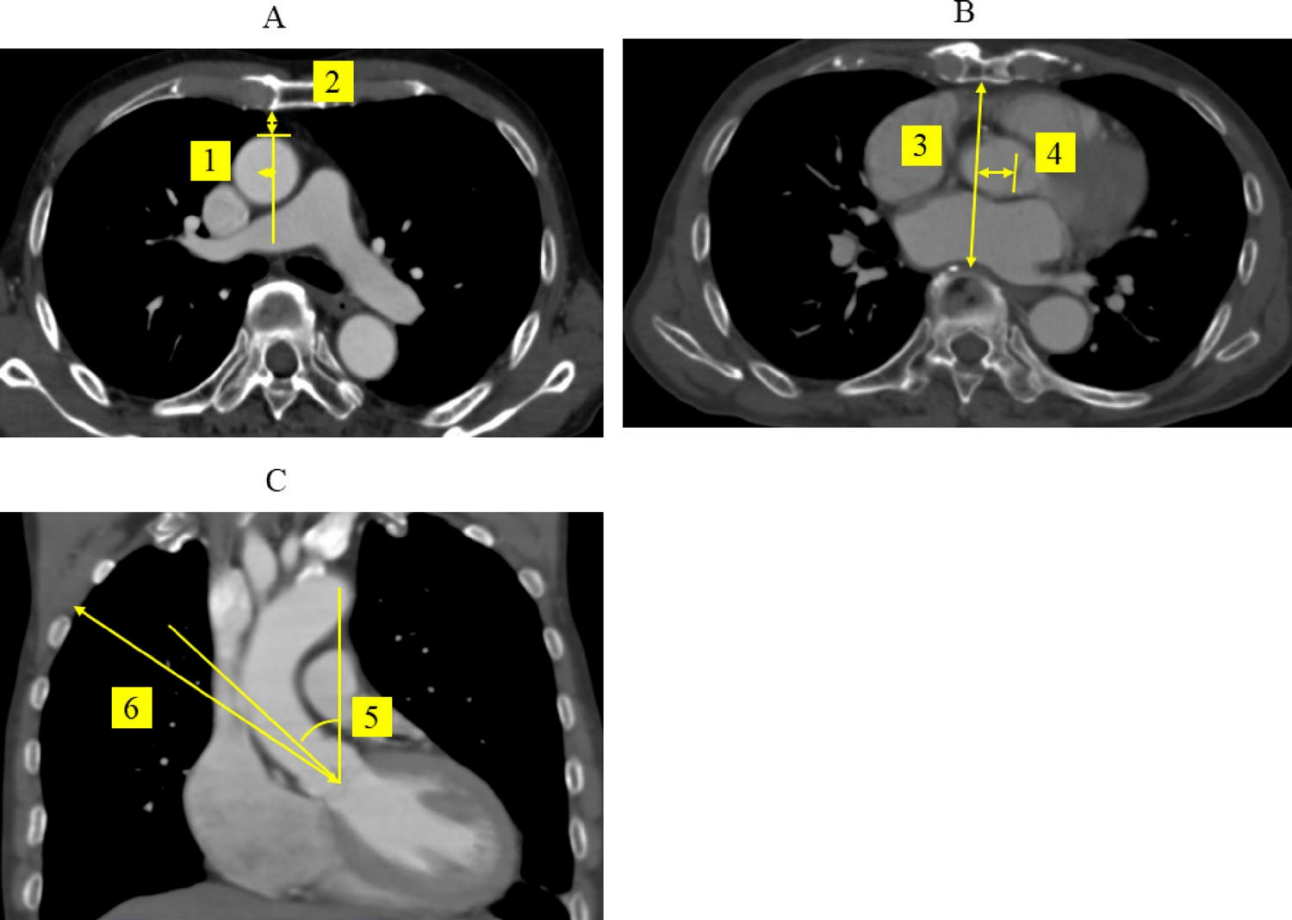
Preoperative CT: (1) laterality of aorta (dextroposition was measured as the distance of the aortic center lateral to the right sterna edge at the same level of the pulmonary trunk on axial image), (2) aorta depth (the distance from the posterior sternal edge to the anterior aortic edge at the level of the pulmonary trunk on axial image), (3) anteroposterior (AP) thoracic diameter (distance from the sternum to the vertebra at the level of the AV at the same level of AV on axial image), (4) AV deviation (distance from the median line to the AV at the same level of AV on axial image), (5) aortic angle (the angle between the ascending aorta and the vertical axis on the coronal image), and (6) the third ICS distance (the distance between the third ICS and the AV on the coronal image)
